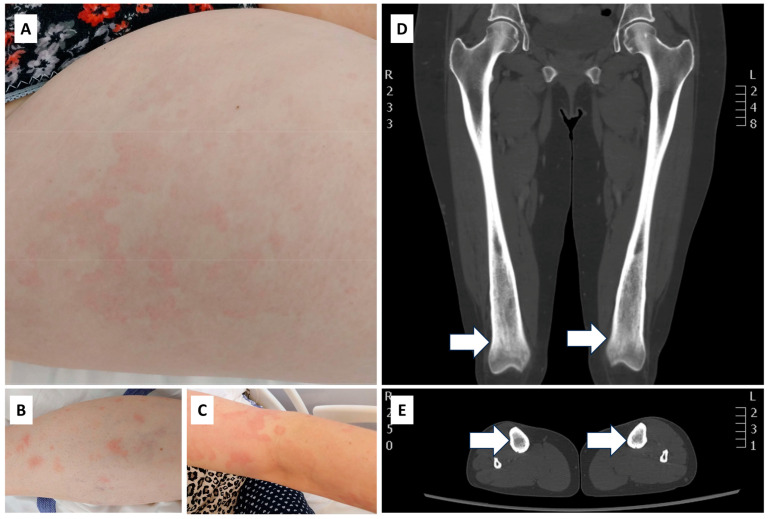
The patient with Schnitzler syndrome has urticarial rashes on her thigh (A), leg (B), and forearm (C). Coronal CT scan of the thighs (D) indicated ill-delineated marrow infiltration (arrows) in the distal metaphyses and epiphyses of both femurs and vague intramedullary sclerosis (arrows) of the proximal tibias (E). L: left, R: right, CT: computed tomography.
